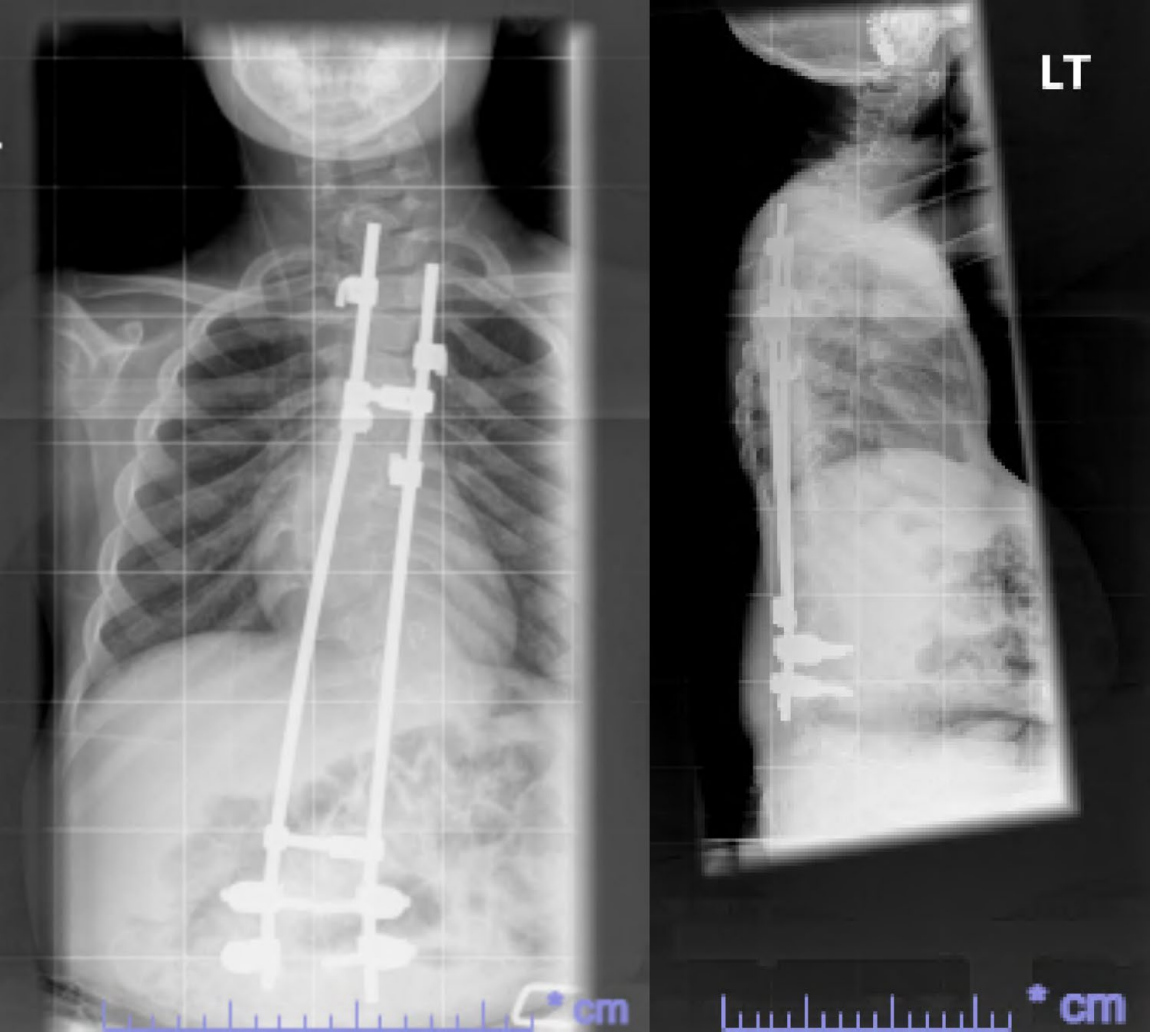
Post operative X-ray after first lengthening of TGR, aged 7
